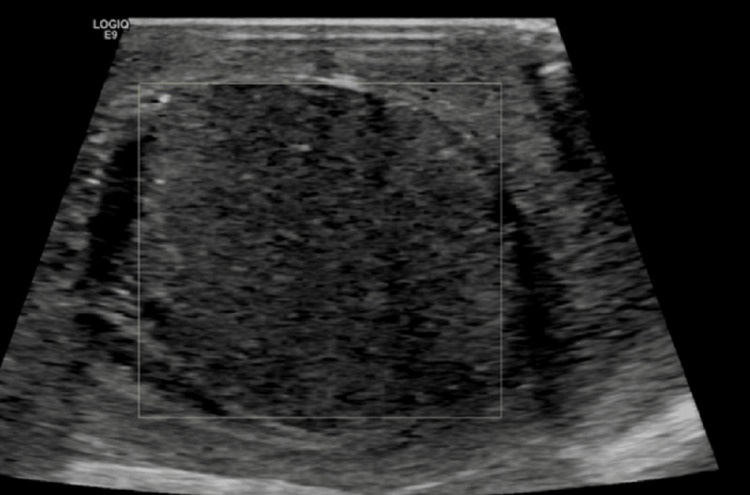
Scrotal Doppler ultrasound of the left testis showing absent blood flow.
